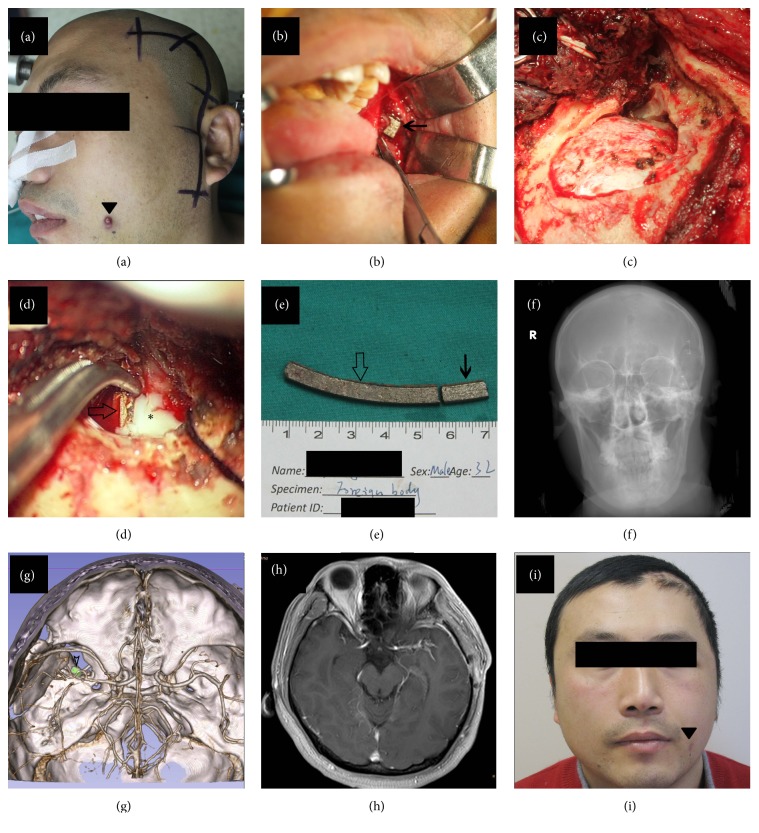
Photography showed the entry point (black triangle, ▼) of foreign body on face and incision for a frontotemporoorbitozygomatic approach (a). Short piece of foreign body (simple arrow, *←*) in the face was exposed and removed by a maxillofacial surgeon (b). The infratemporal fossa was opened to expose the long piece of foreign body (c), and it (hollow arrow, ⇦) was removed in a retrograde fashion. During the removing process, yellowish pus (asterisk, *∗*) was drained into the infratemporal fossa (d). Photography displayed short piece (simple arrow, *←*) and long piece (hollow arrow, ⇦) of the foreign body (e). Postoperative skull radiography suggested complete removal of the foreign body (f). The small bone fragment (hollow arrow head, *➤*) was left in place due to its close relationship with branch of MCA (g). Three-month follow-up MRI revealed no abscess formation (h). The patient recovered uneventfully (i). MCA, middle cerebral artery; MRI, magnetic resonance imaging.
